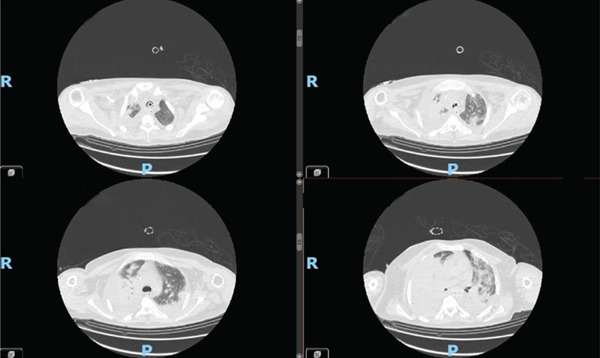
CT scan performed 10 days after admission.
